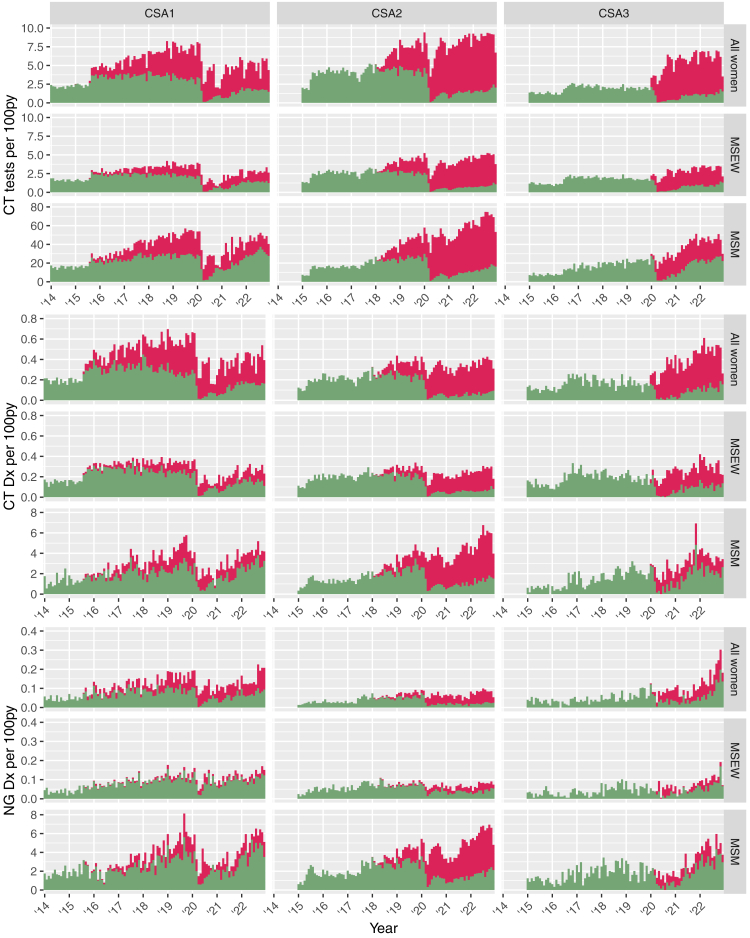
Monthly incidence rates of chlamydia (CT) + gonorrhoea (NG) testing activity and diagnoses of CT and NG. Columns correspond to the three Case Study Areas (CSA1-3), and data for each outcome are disaggregated by sex and sexual behaviour: women, men who have sex exclusively with women (MSEW) and men who have sex with men (MSM). Test performed in clinic are shown in green and online postal self-sampling (OPSS) tests are shown in red.
